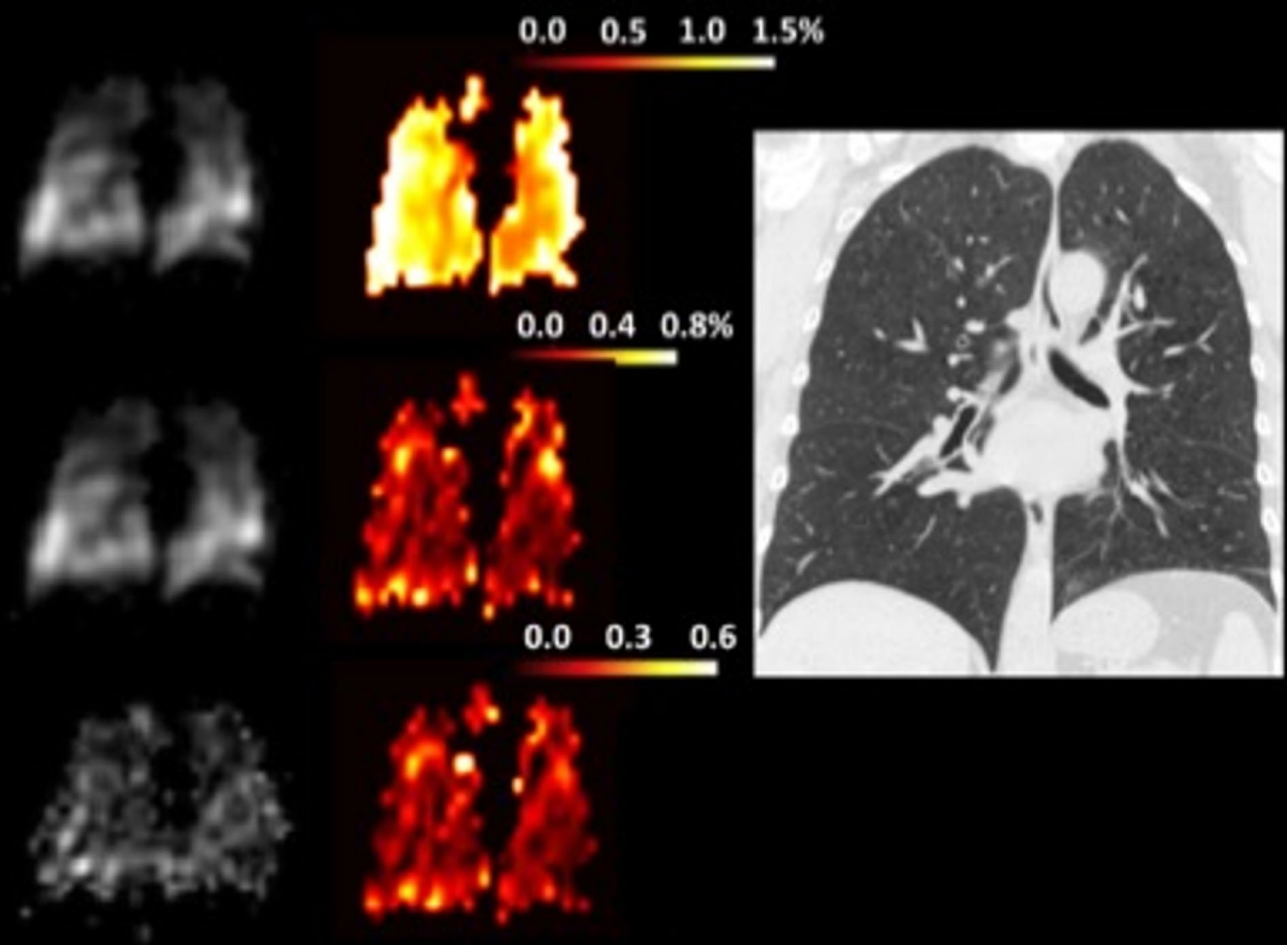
High resolution CT and hyperpolarized ^129^Xe dissolved-phase MR images in patient with COPD. In order, image reconstructions of gas (top left), tissue (left middle) and red blood cells (bottom left) compartments are presented. The paired color gradient images are fused reconstructions demonstrating relative gas content in the different compartment; tissue/gas, RBC/gas and RBC/tissue. Adapted from [16] with permission
